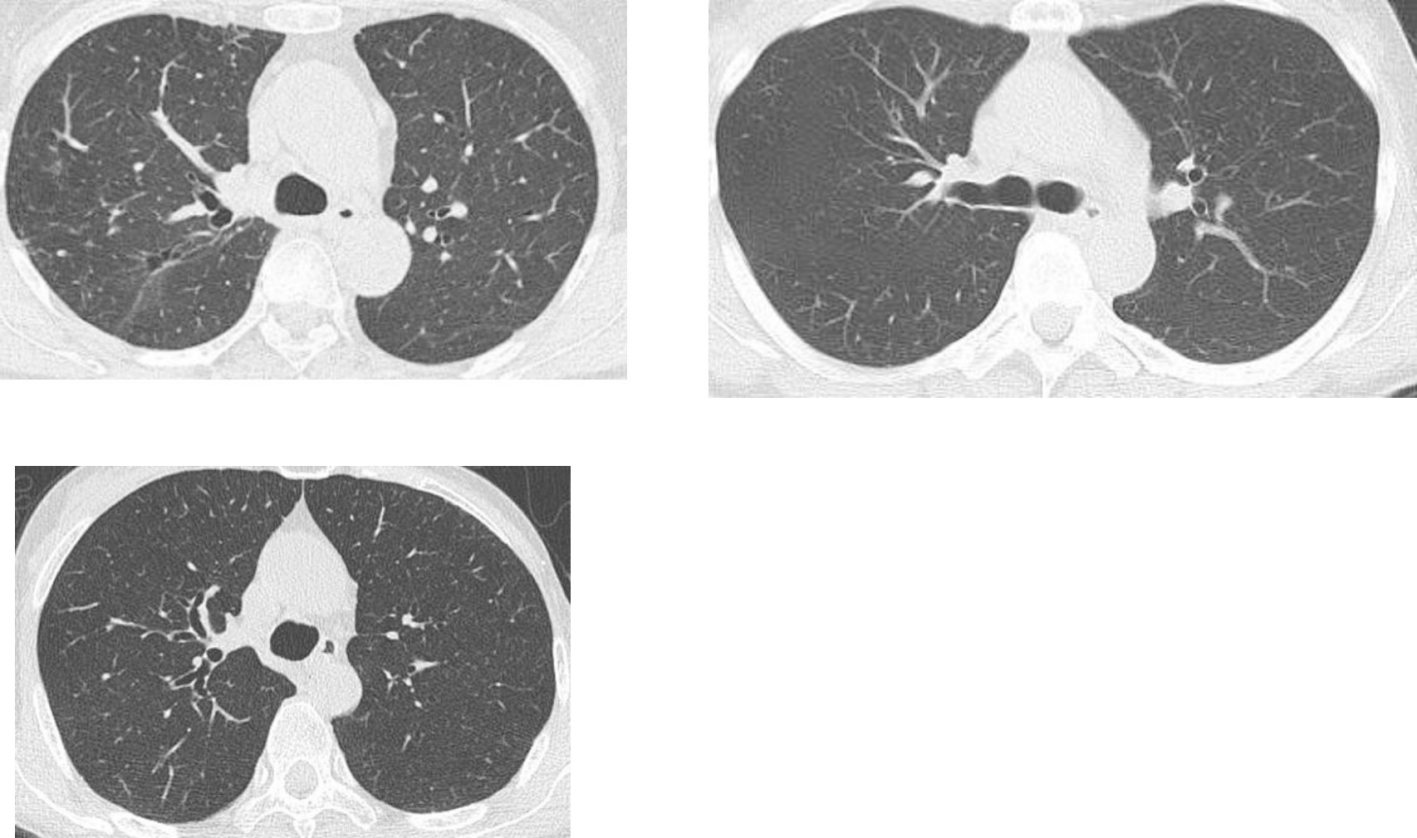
Typical cases of normal esophageal diameter. CT images of three cases whose maximum diameter of esophagus is less than 10 mm were shown.
